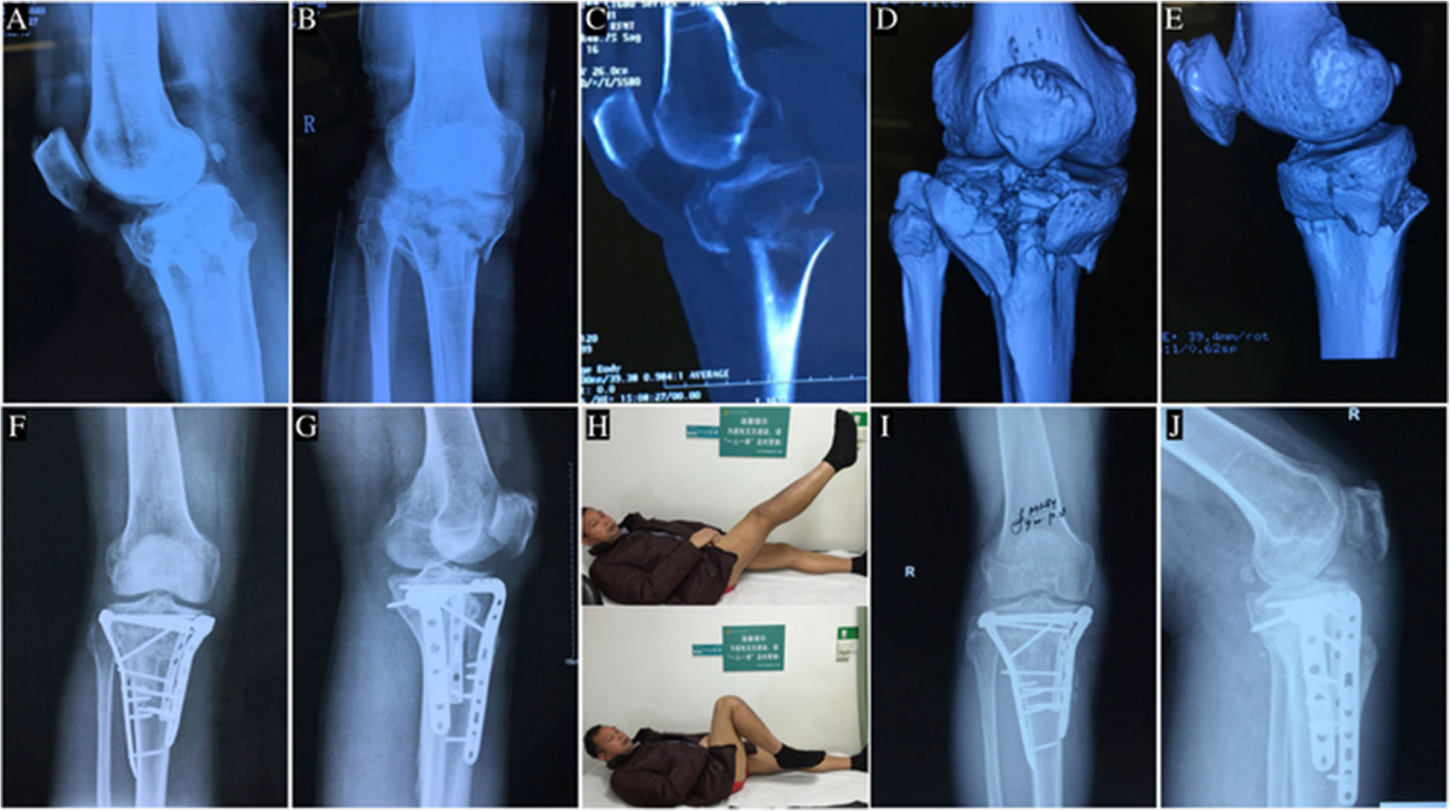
A 48-year-old man with hyperextension bicondylar tibial plateau fracture treated with anterolateral and posteromedial double incisions. a Preoperative anteroposterior view of X-ray. b Preoperative lateral view of X-ray shows a reversed pTSA. c,d,e Three-dimensional reconstruction CT shows collapse of the anterior part of tibial plateau and a large displacement of the posterior tibial cortex. f,g Postoperative anteroposterior view and lateral view of X-ray. h The function of the knee has recovered at the 9-month follow up. i,j Anteroposterior view and lateral view of X-ray show the fracture has healed at the 9-month follow up
